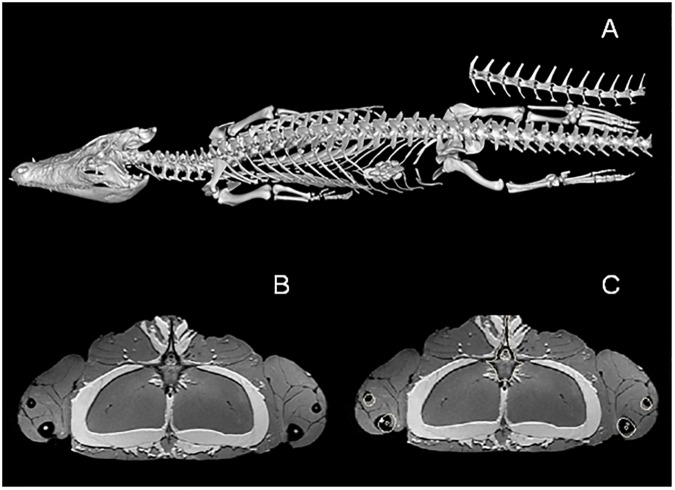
Combining CT and MRI data. CT and MRI data of *Crocodylus porosus* (XCb Cp4). A = skeleton extracted from CT; B = MRI coronal view of upper hindlimbs; C = same images as B with bone outlines from CT included.
